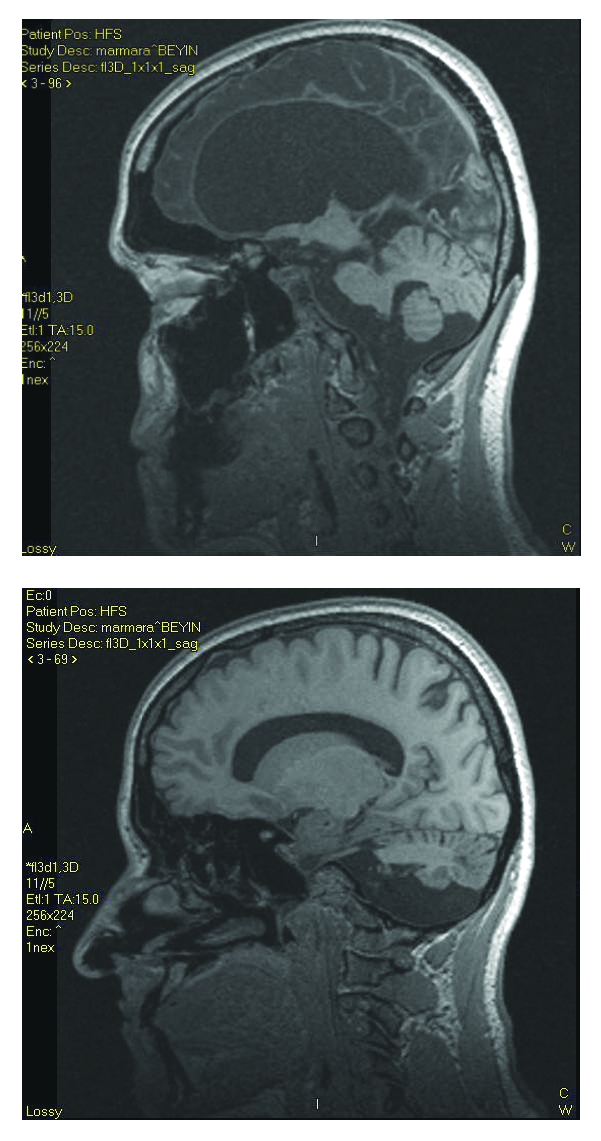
Cranial MRI shows total left hemisphere encephalomalasia which looked like the previous cranial CT
